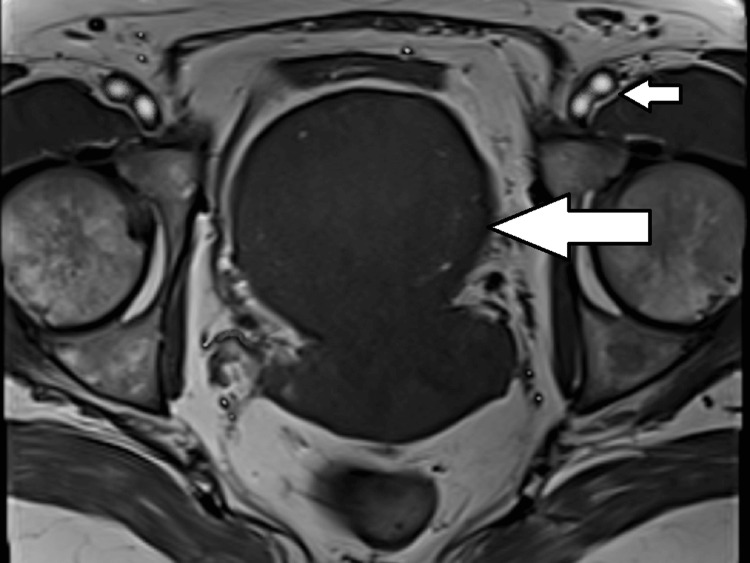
Post contrast T1-weighted axial section of the pelvis shows no enhancement of the lesion (large arrow) in comparison to the enhancing iliac vessels (small arrow).
